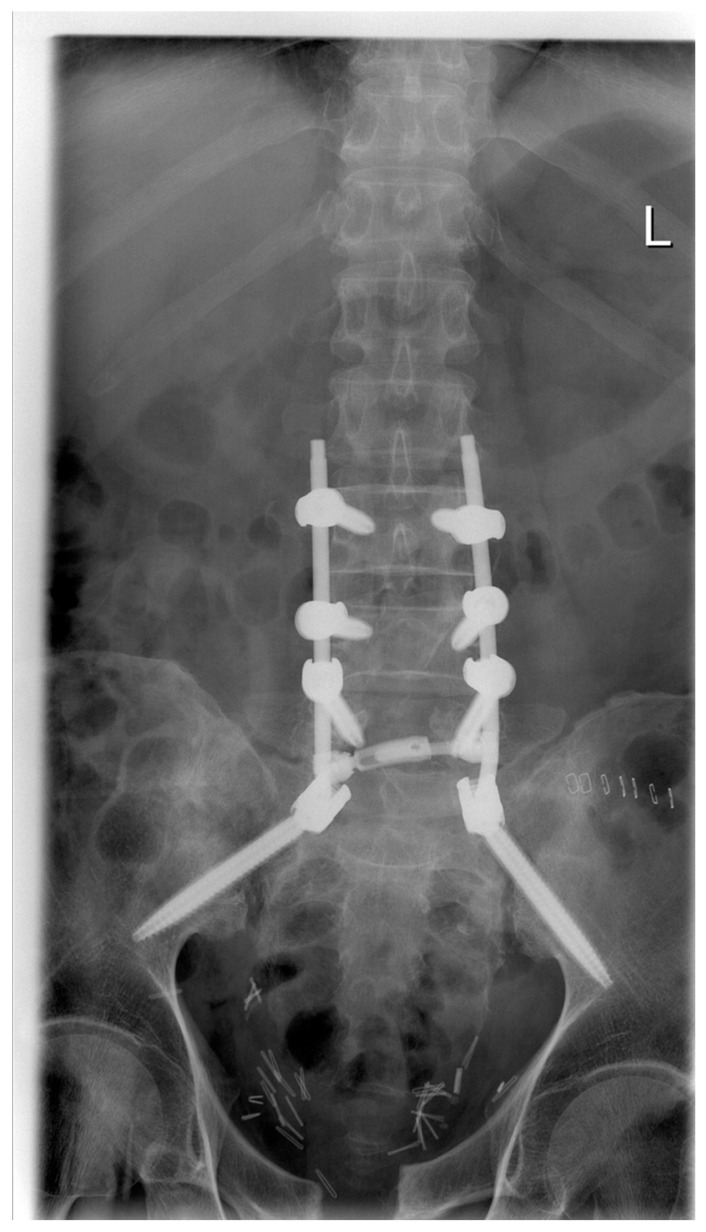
Showing the same patient as in Figure 4 in a coronal standing X-ray following loosening of implants, removal, and restabilization via spinopelvic stabilization (Stryker, K2M, EVEREST^®^) L3–S2–Ala–Ilium.
